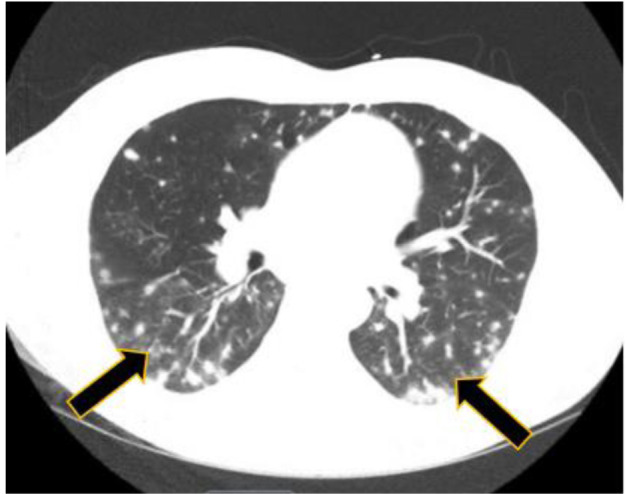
Patient 1 CT scan of upper chest with evidence of diffuse granulomatous disease
